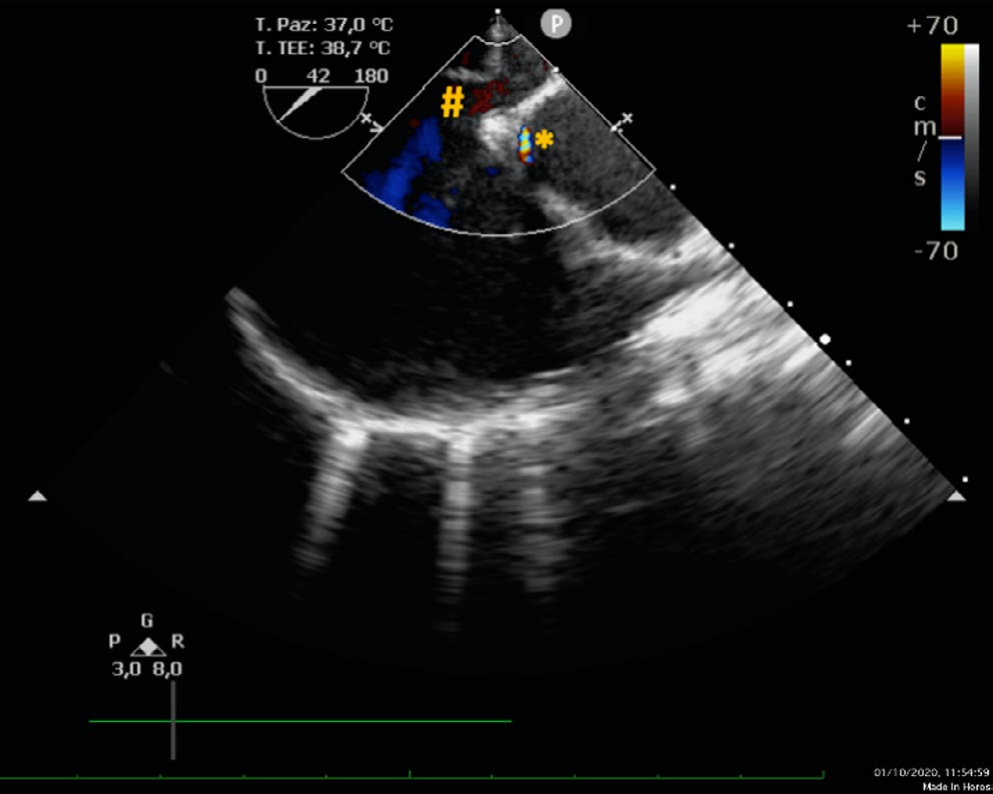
Aortic short axis transesophageal echocardiography showing main atrial septal defect (hash) and an unusual flow behind the noncoronary aortic sinus (asterisk)
